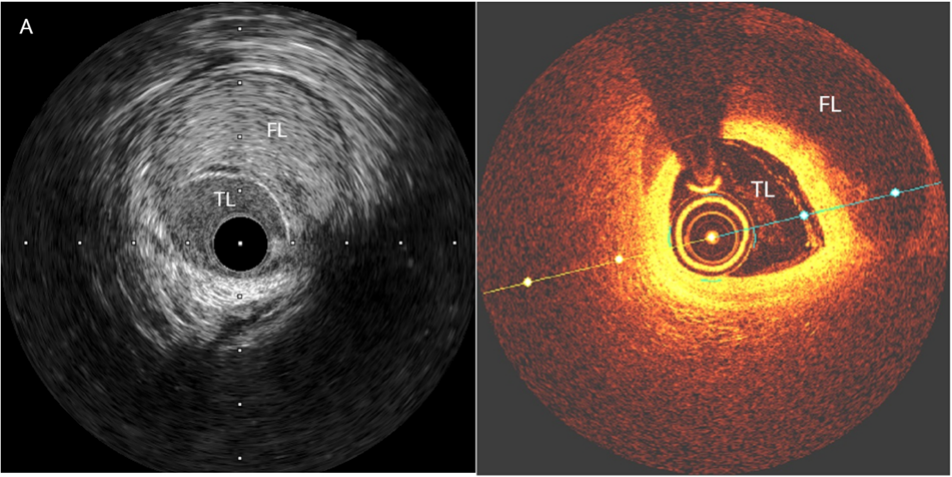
Intracoronary imaging findings of spontaneous coronary artery dissection. A intravascular ultrasound image of SCAD; B Optical coherence tomography image of SCAD. Images sourced from the ANZ-SCAD Registry^83^. SCAD spontaneous coronary artery dissection, FL false lumen, TL true lumen.
